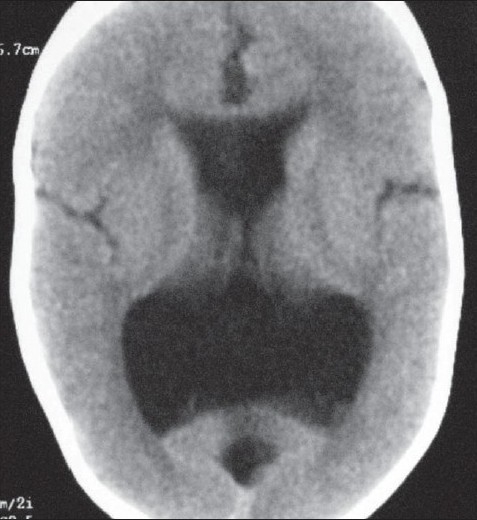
Noncontrast axial CT scan showing the presence of colpocephaly with septal agenesis
